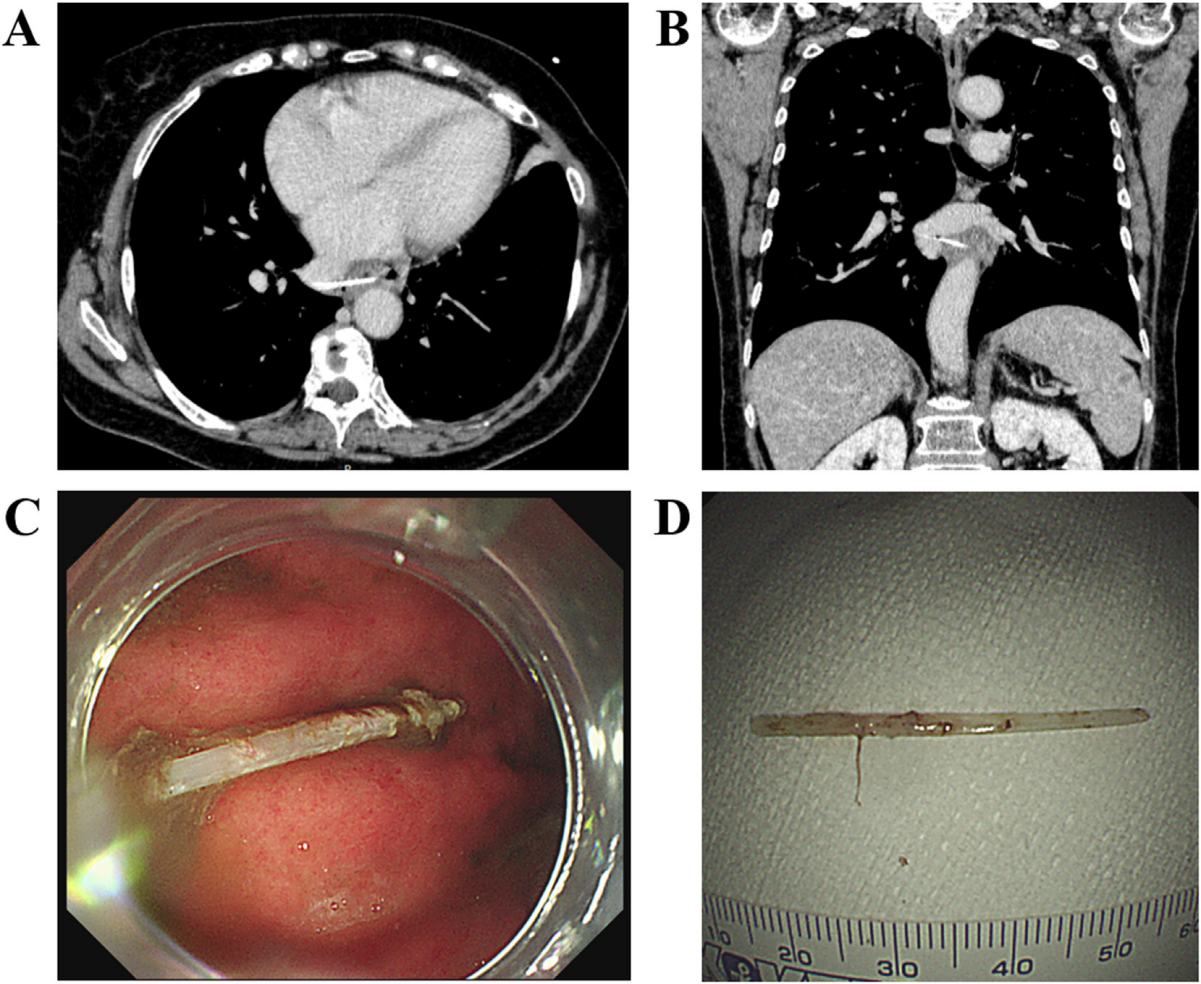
Contrast-enhanced chest computed tomography images and endoscopic findings on bone removal. The axial view (A) and the coronal view (B) of the chest computed tomography scan with a slice thickness of 1 mm revealed a fish bone of 35 mm in length. It penetrated from the right side of the middle intrathoracic esophagus into the right inferior pulmonary vein, accompanied by a small amount of air bubbles. Slice thickness: 1 mm, window level: 50 HU, window width: 300 HU. (C, D) Endoscopic finding on the bone removal, showing the impacted bone with 35 mm length.
